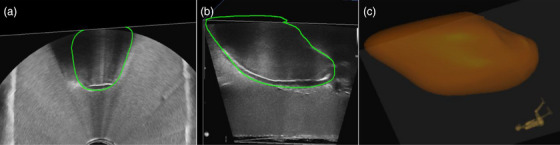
(a,b) TRUS contour of the uterus (green) displayed in the transverse (a) and sagittal (b) planes; (c) resulting in 3D‐rendered uterine volume. TRUS, transrectal ultrasound.
